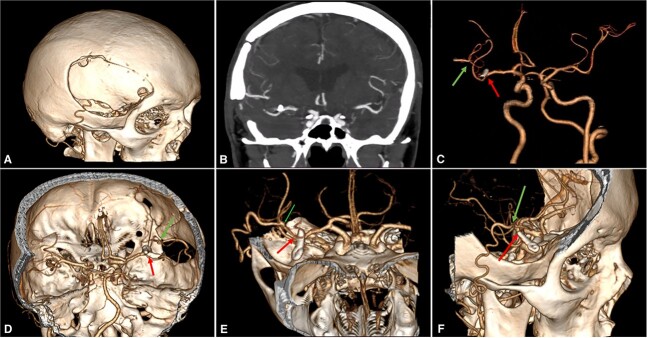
Postoperative Imaging. (A) 3-Dimensional image reconstruction shows the craniotomy with the entrance of the STA into the skull; (B) Coronal view of a maximum intensity projected multislice spiral CT shows the permanent clip placed with the exclusion of the fusiform aneurysm; (C)–(F) Cranial views depict patency of the STA-MCA bypass (green arrow) and clipping of the M2 proximal segment (red arrow). CT: computed tomography, MCA: middle cerebral artery, STA: superficial temporal artery.
